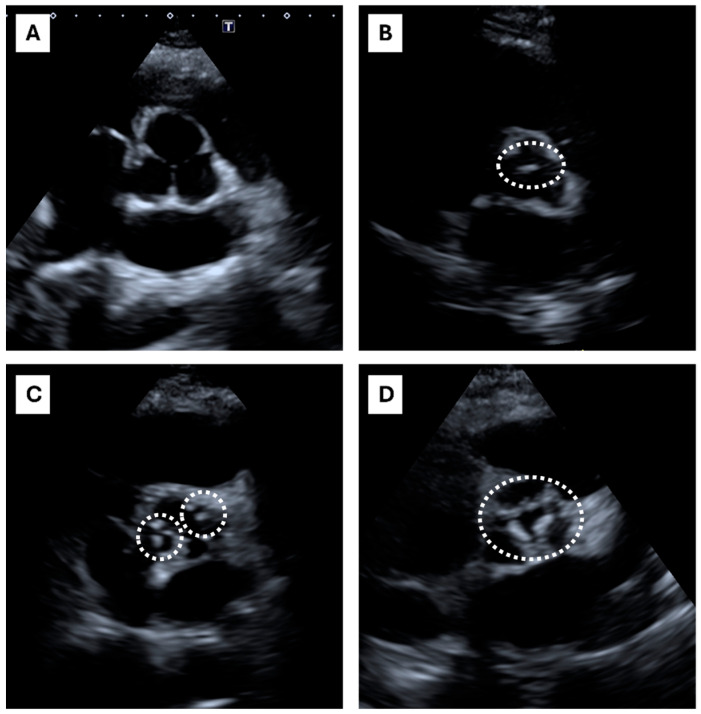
Echocardiographic calcium score: echocardiographic short-axis parasternal view. The figure shows the echocardiographic short-axis parasternal view, focused on the aortic valve. At the center of each box, aortic valve and cusps are shown. The dashed lines encircle aortic valve calcifications. The ECS scoring system considers four classes: (A) ECS = 0, Absent (no calcifications); (B) ECS = 1, Mildly calcified (the dashed line encircles an isolated small spot), (A,C) ECS = 2, Moderately calcified (multiple bigger spots are encircled by dashed lines), (D) ECS = 3, Heavily calcified (extensive thickening/calcification of all cusps).
